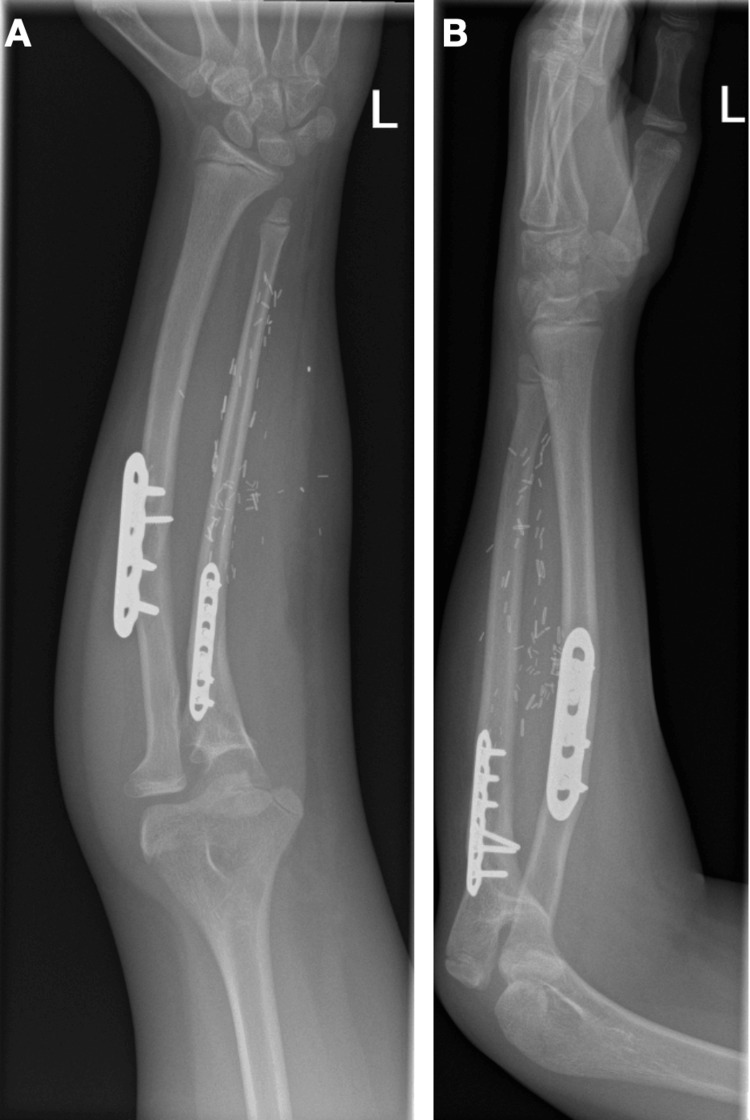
(A) AP and (B) lateral X-rays of the patient’s left forearm showing the transposed fibula to the left ulna AP, anteroposterior
